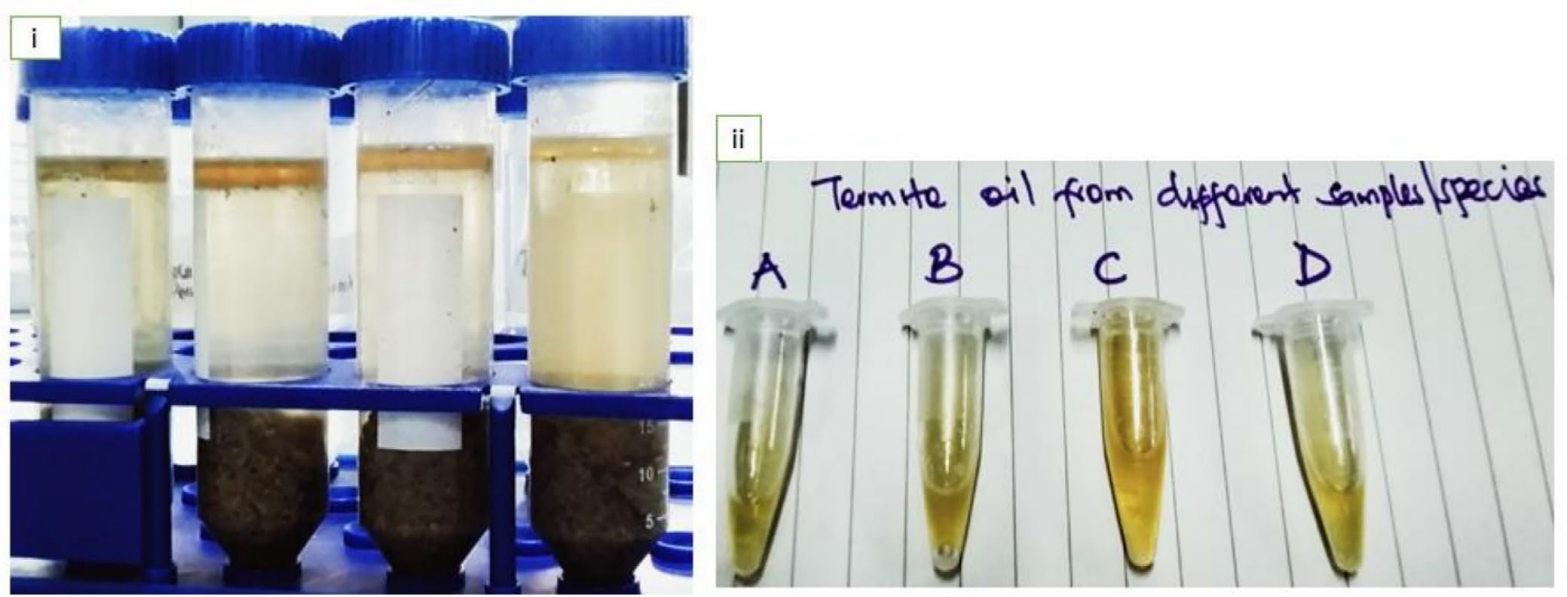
Ultrasound‐assisted termite oil extraction, with an ethanol‐isopropanol solvent; (i) Termite flour‐solvent suspensions upon settling, (ii) samples of recovered oil after sonication.
